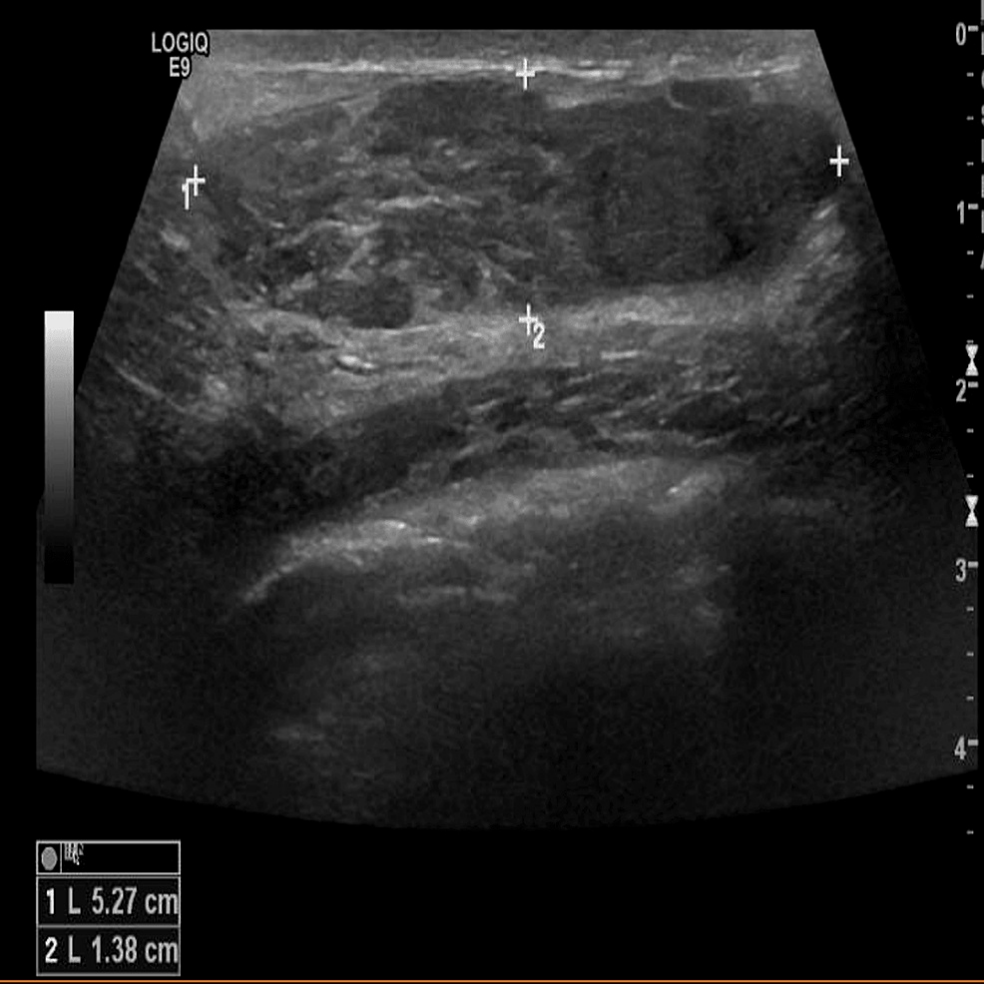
Right breast USS at 12 weeks showing a considerable increase in the size of previous lesion to 91 mm. USS, ultrasound scan
